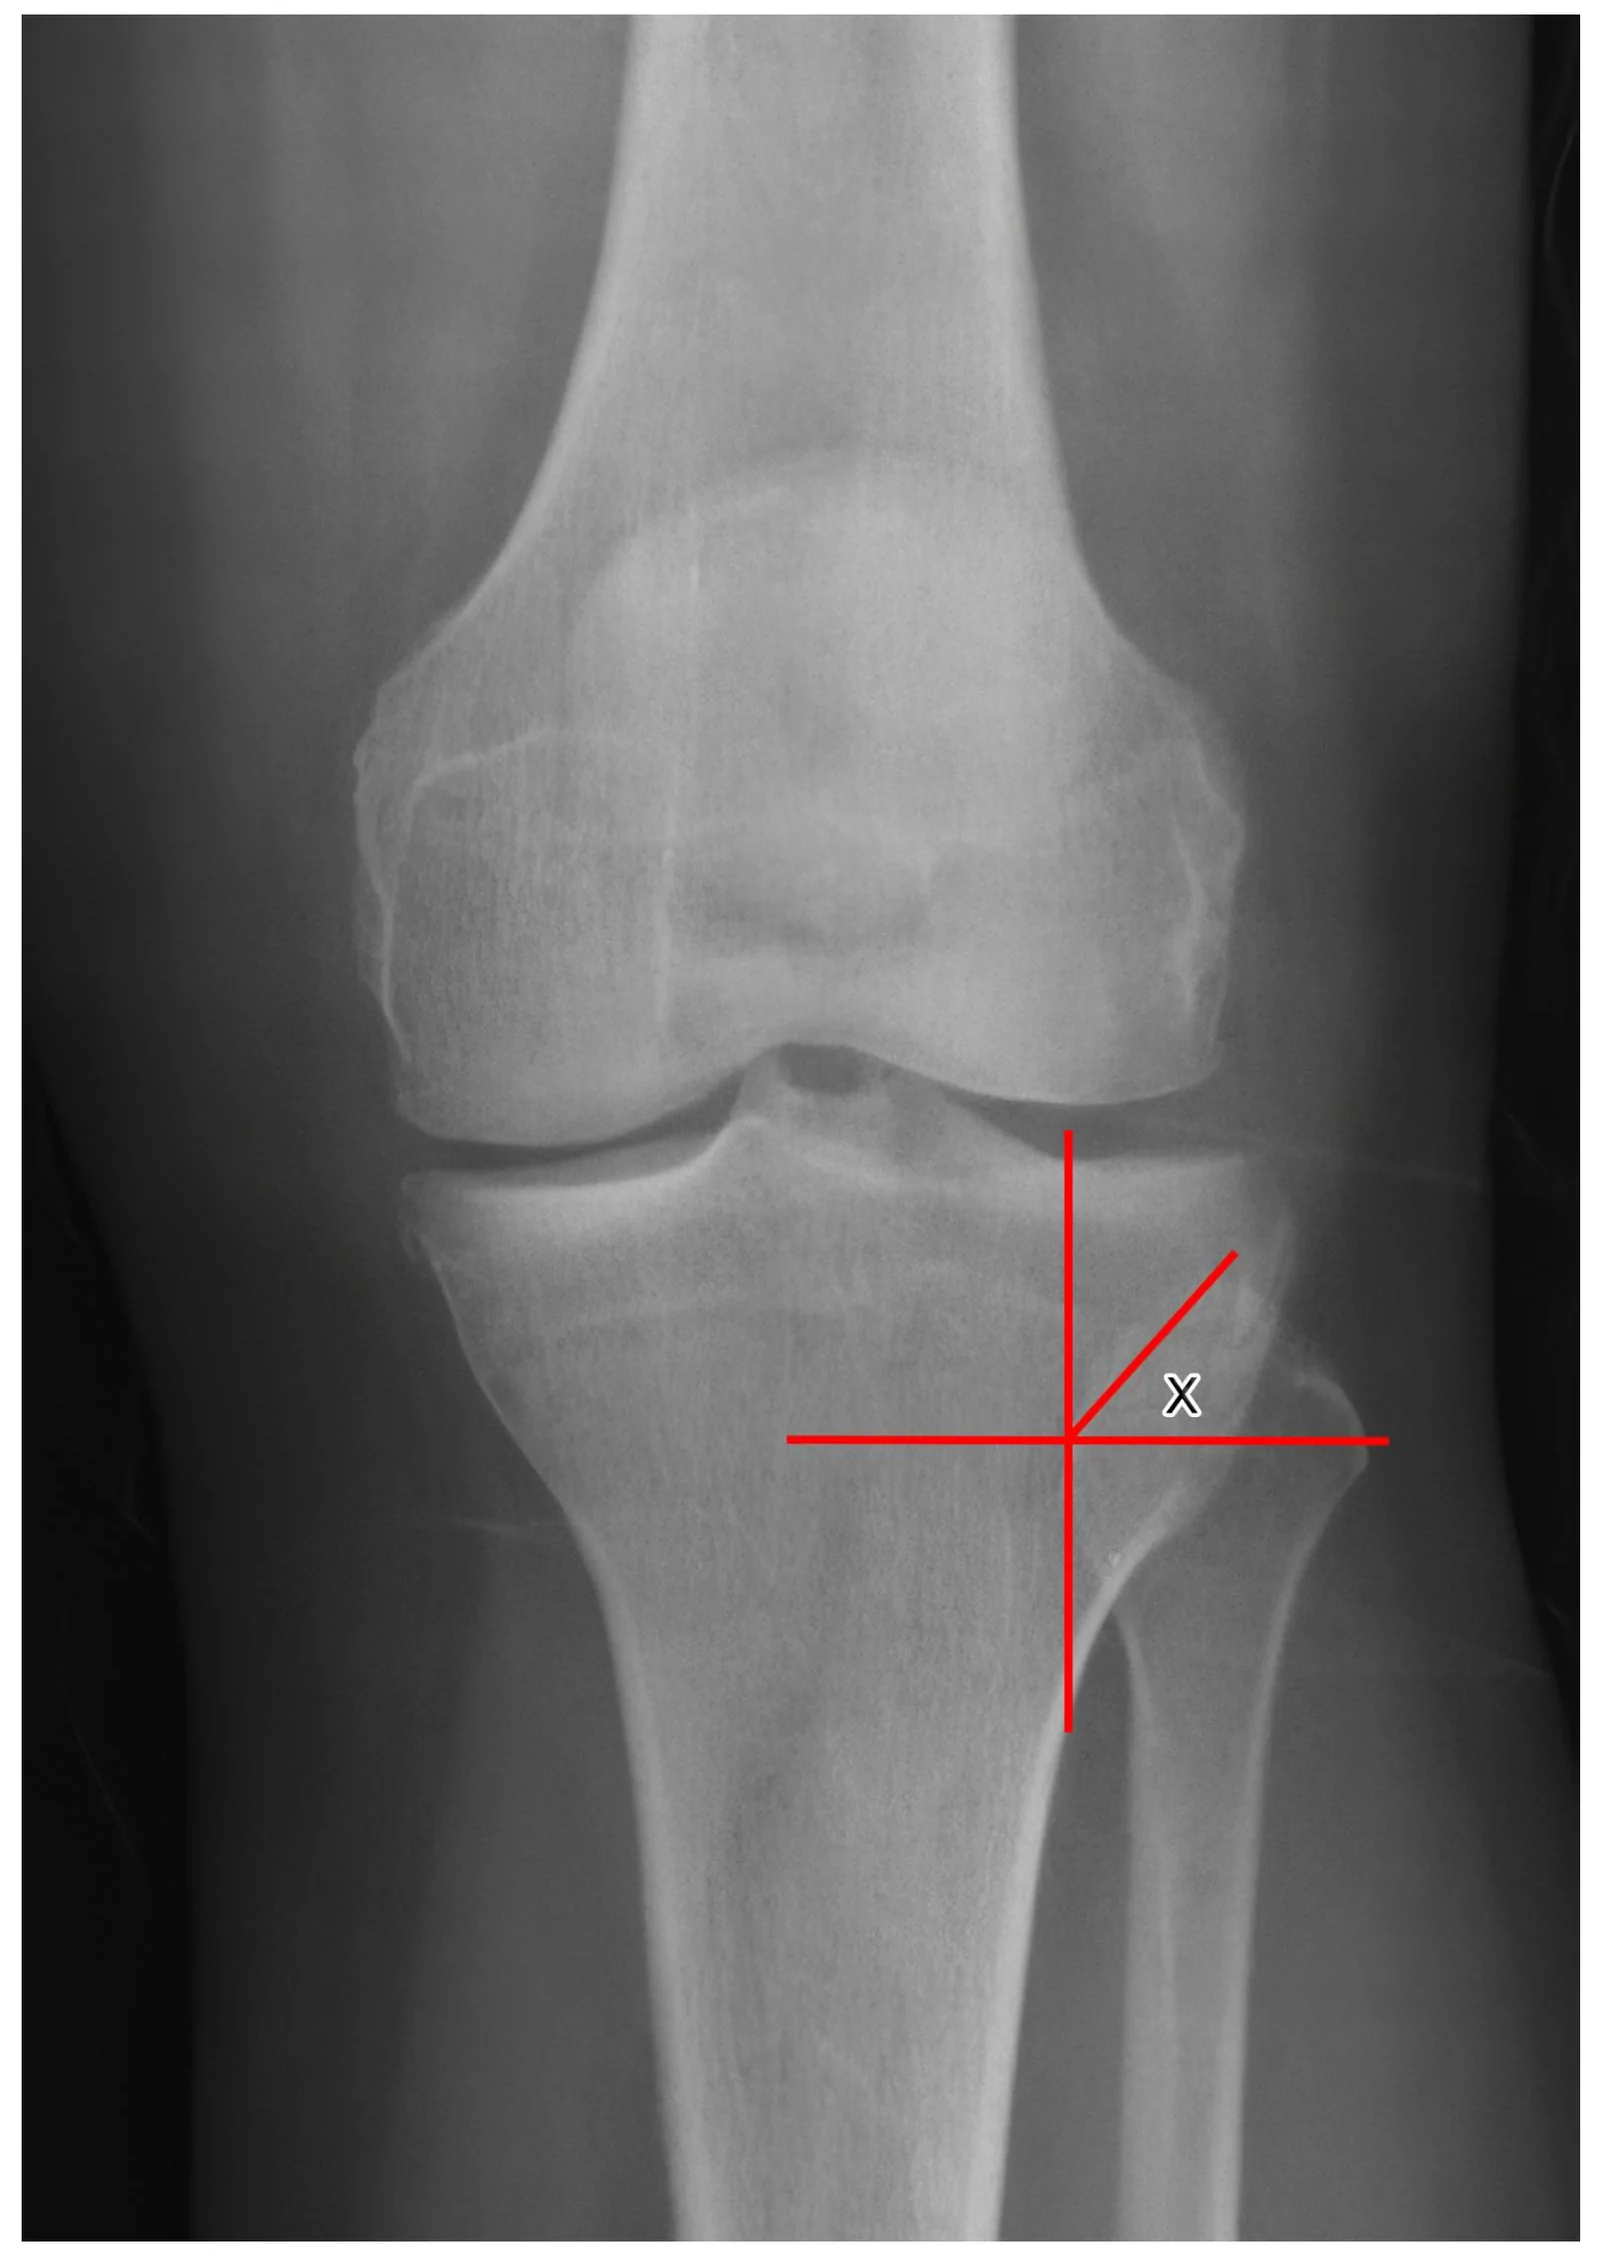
Measurement of the proximal fibular articular-surface inclination angle in the anteroposterior radiographic view. The angle was determined by constructing three reference lines: the first line was drawn tangentially along the medial cortex of the fibular diaphysis; the second line was drawn perpendicular to the first, with its intersection positioned at the most inferior portion of the proximal tibiofibular joint; and the third line originated at the apex of the fibula and was drawn tangentially along the medial surface of the fibular head. The angle between the second and third lines represents the proximal tibiofibular joint inclination angle.
